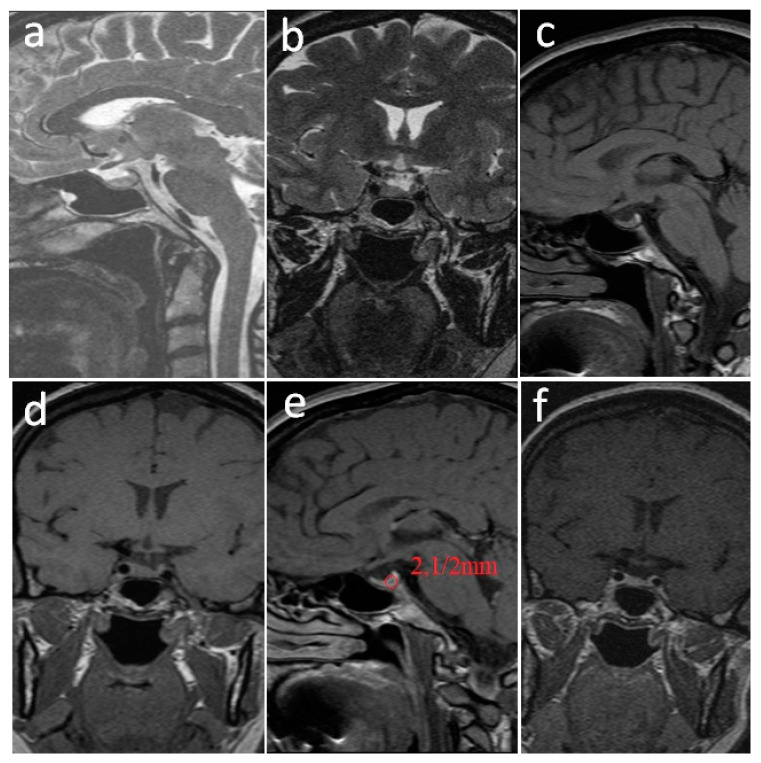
Pituitary MRI performed 4 years later when the patient was 6 weeks pregnant. (a). Non-contrast sagittal T2-weighted image. (b). Non-contrast coronal T2-weighted image. (c). Non-contrast sagittal T1-weighted image. (d). Non-contrast coronal T1-weighted image. (e). Post-contrast sagittal T1-weighted image. (f). Post-contrast coronal T1-weighted image. Favorable evolution of the pituitary adenoma located in the left parapituitary in moderate high-signal T2-weighted (a,b), low-signal T1-weighted (c,d), and non-gadolinium enhanced (e,f) with dimensions reduced to 2.1/2 mm.
